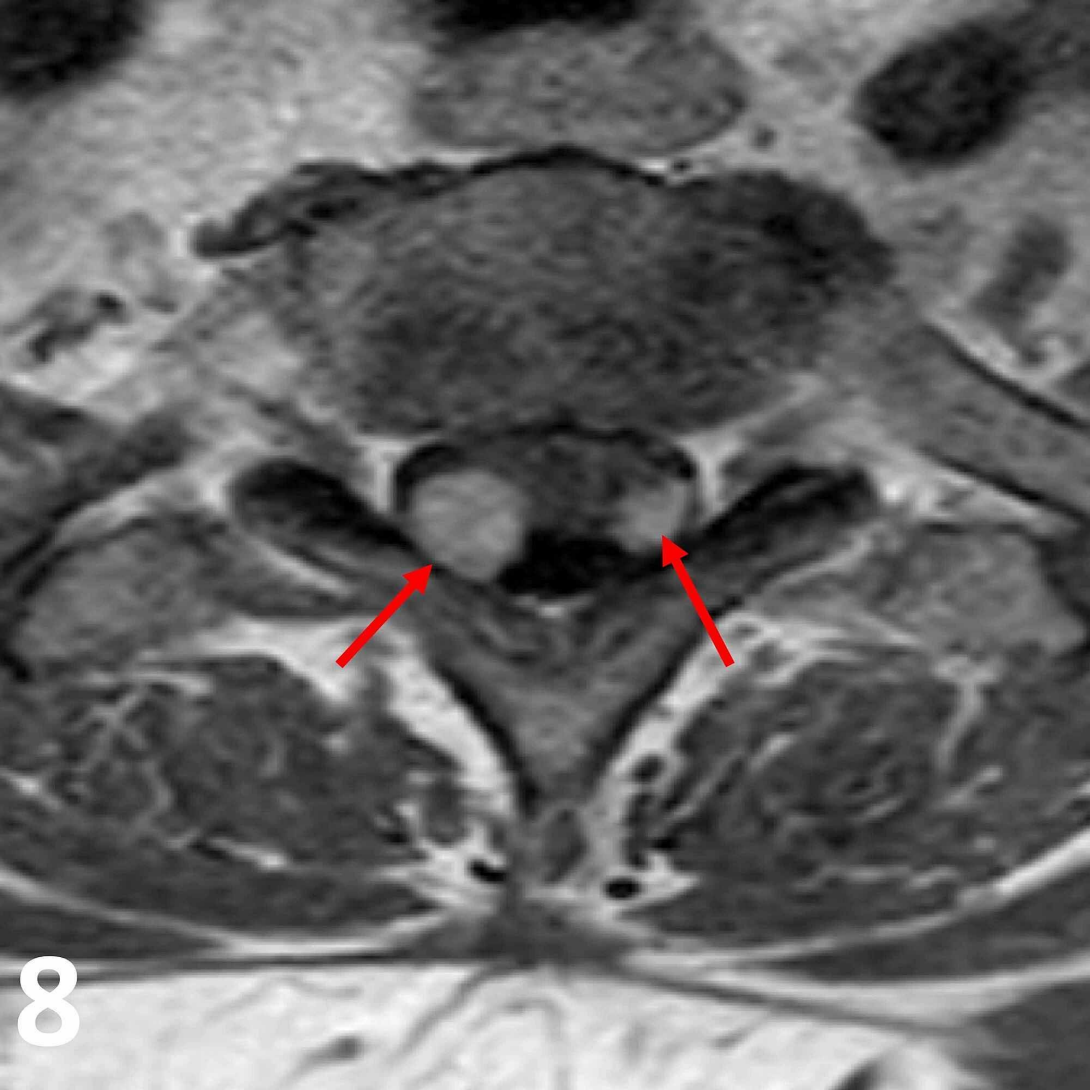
Axial T1 post-contrast MRI image at the T1/T2 vertebral level. Note the homogenously enhancing intradural lesions (red arrows) in the right posterolateral aspect of the spinal cord. Both lesions exhibit intramedullary and extramedullary components, which are unusual and rare for schwannomas.
